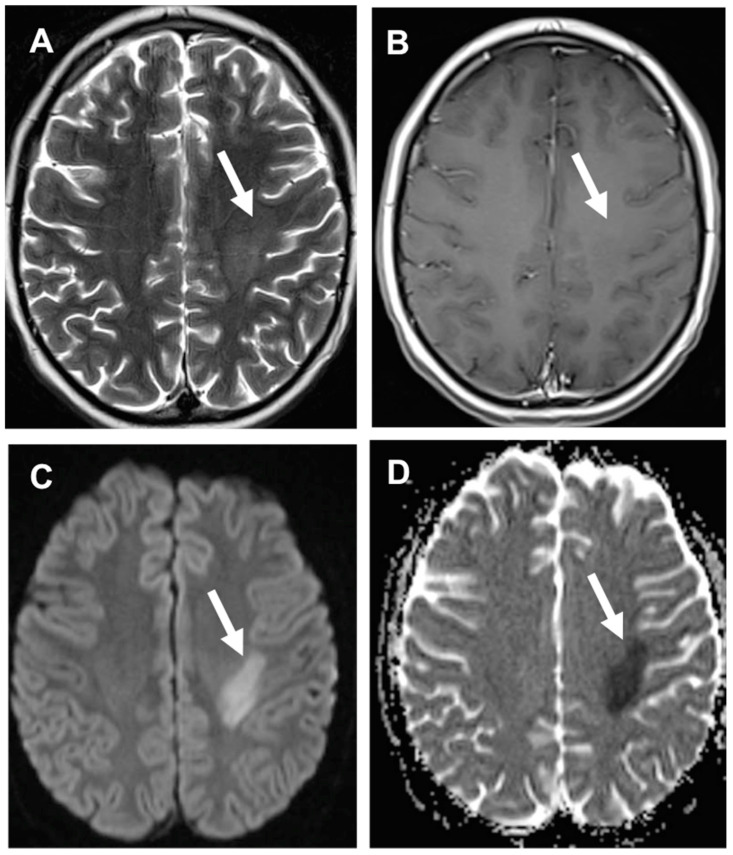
Methotrexate -Induced Neurotoxicity during childhood acute lymphoblastic leukemia (ALL) therapy. Axial T2 w (A) shows an asymmetric area of hyperintense signal in the left corona radiata/centrum semiovale (arrow) with restricted diffusion on DWI (C) and ADC map (D), and no enhancement after gadolinium administration (B).
